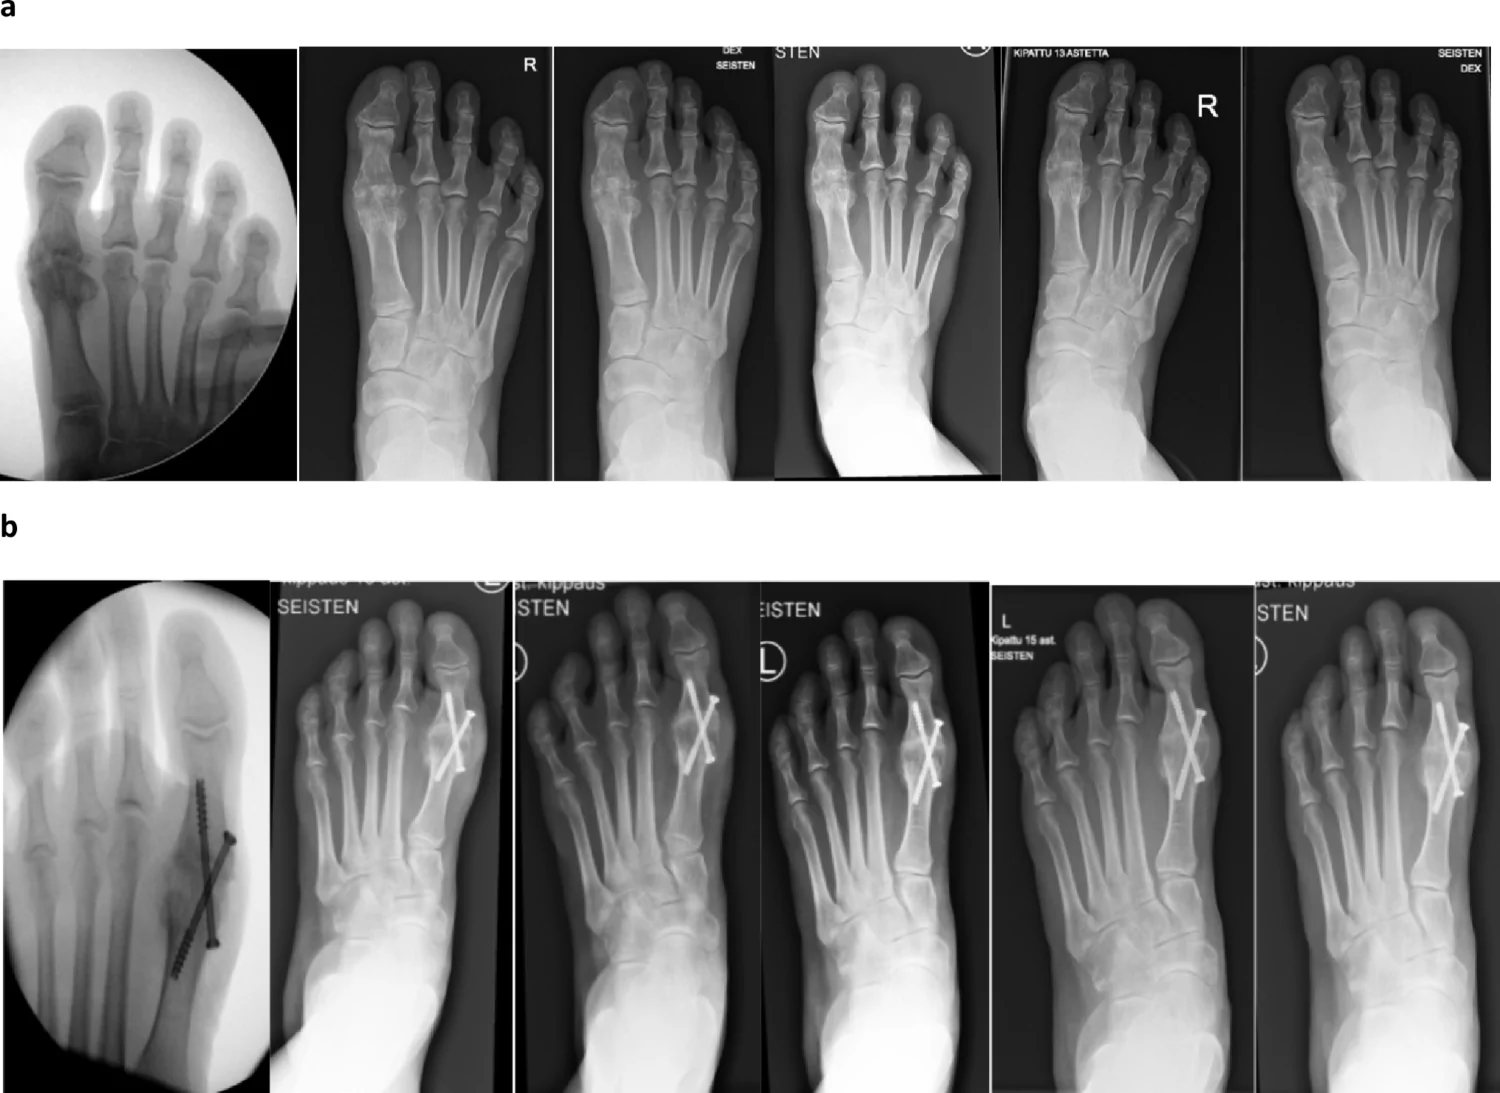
a Bioabsorbable screw fixation. Intraoperative image and follow-up radiographs at 6 weeks, 3 months, 6 months, 12 months, and 24 months. b Titanium screw fixation. Intraoperative image and follow-up radiographs at 6 weeks, 3 months, 6 months, 12 months, and 24 months
